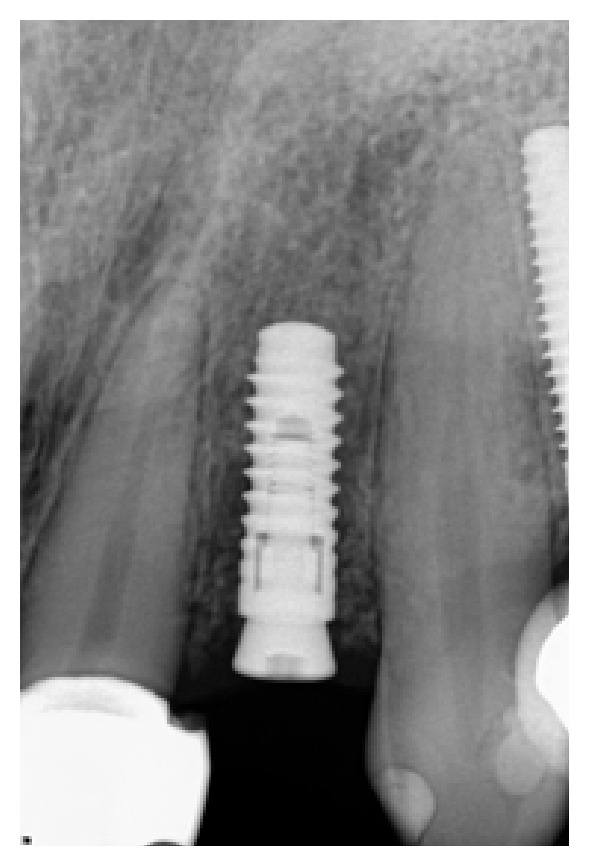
PA radiograph 4 months following bone grafting around the implant.
